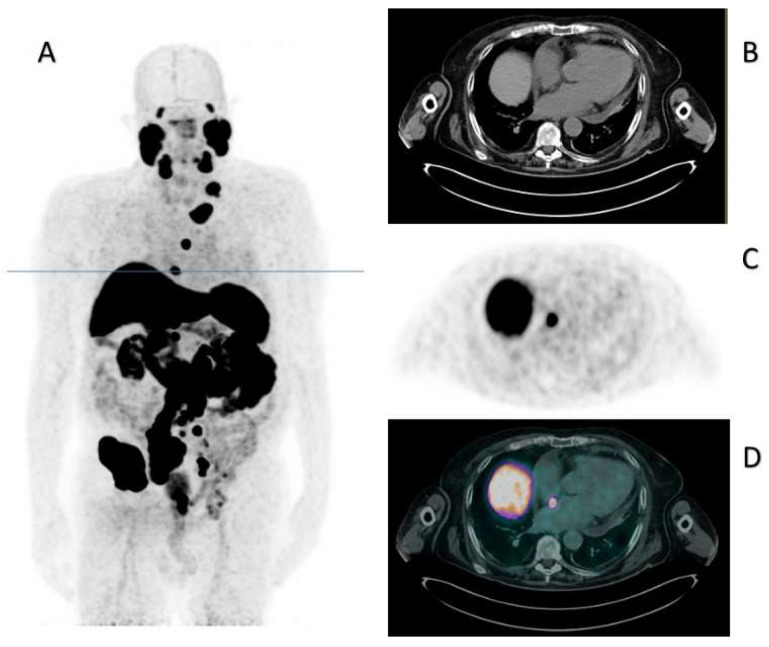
PSMA PET/CT of October 2022. Panel (A) displays the maximum intensity projection (MIP), where the blue line indicates the slice corresponding to the cardiac metastasis. Panels (B–D) indicate the CT, PET, and fused PET/CT corresponding axial images.
